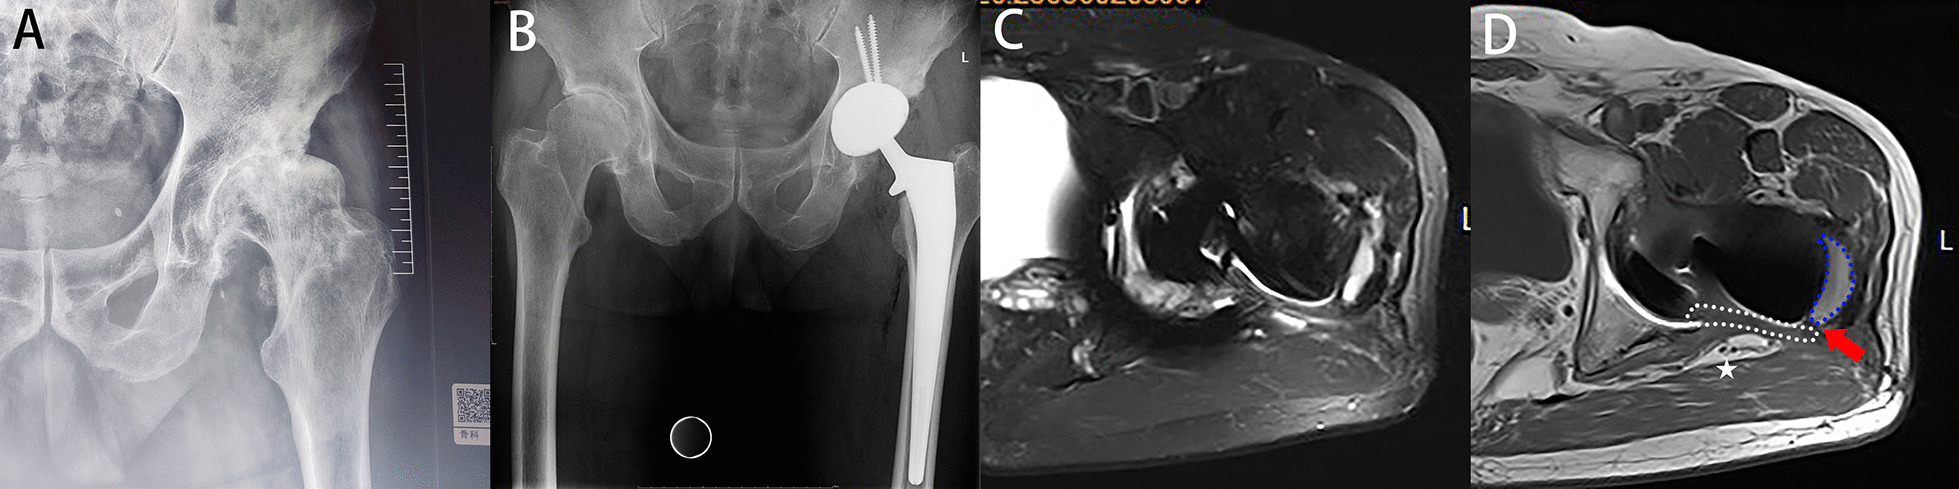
Male, 73 years old, hip osteoarthritis (A). THA with conventional capsular repair was performed (B). Follow-up MRI (C, D) showed the capsule was contacted with the greater trochanter. Symbols are identified as above
